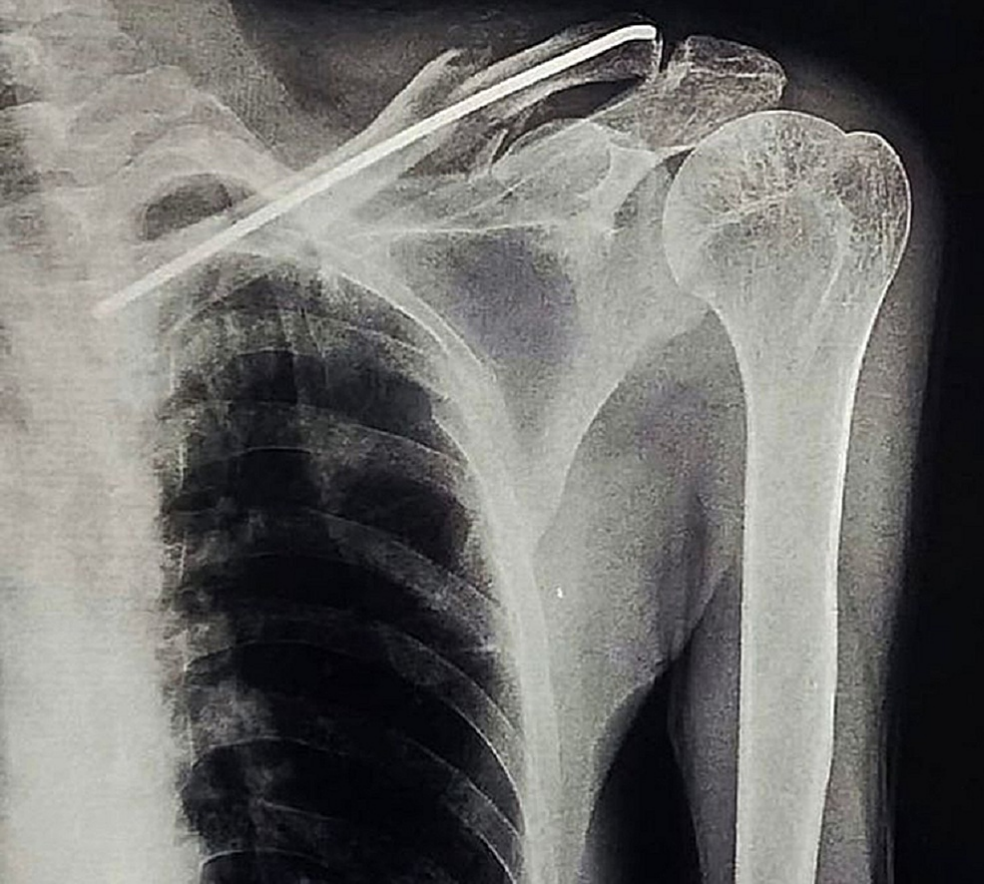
Postoperative X-ray of left clavicle fracture treated with intramedullary fixation using a titanium elastic nail (TEN)
